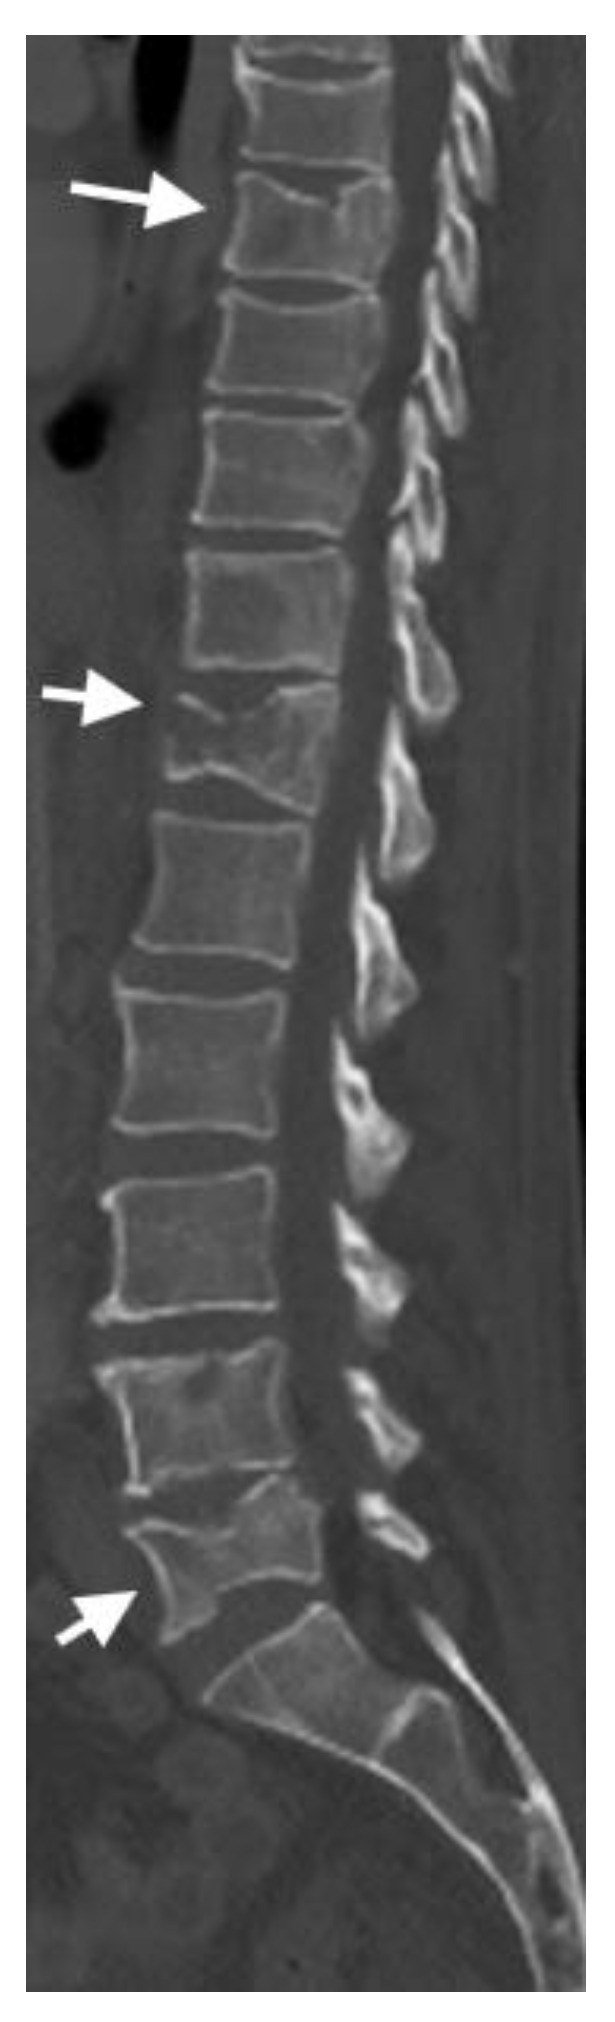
In this sagittal CT, the arrows show multifocal lytic lesions throughout the thoracolumbar spine. Multilevel, mild, chronic compression deformities of several vertebrae in this patient with metastatic melanoma.
